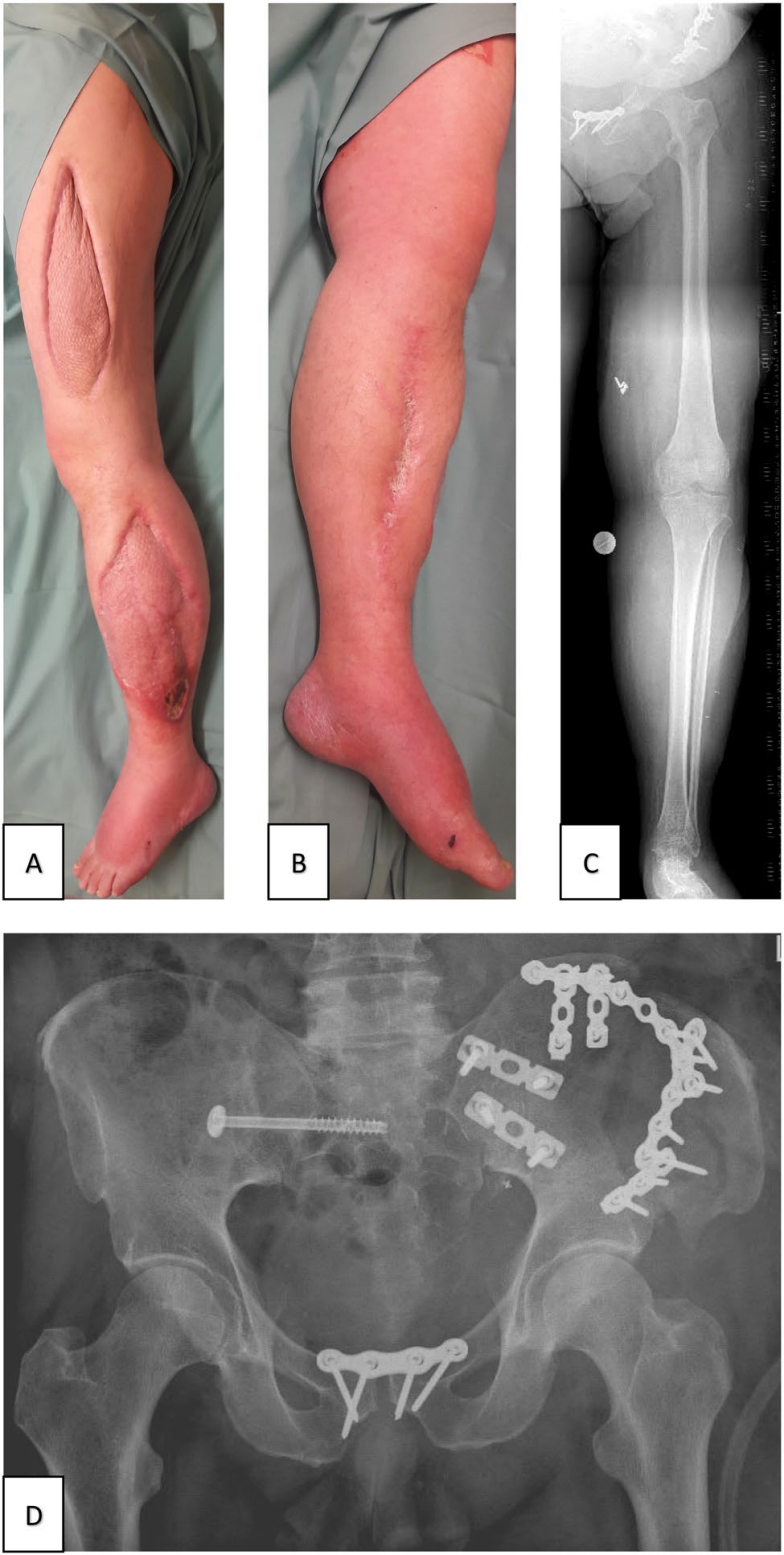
Patient 3 follow-up 14 months after the accident. A, B, C Clinical images and radiograph of the salvaged limb with a split-skin graft of fasciotomy. D Radiograph of the pelvis 4 months after the accident
